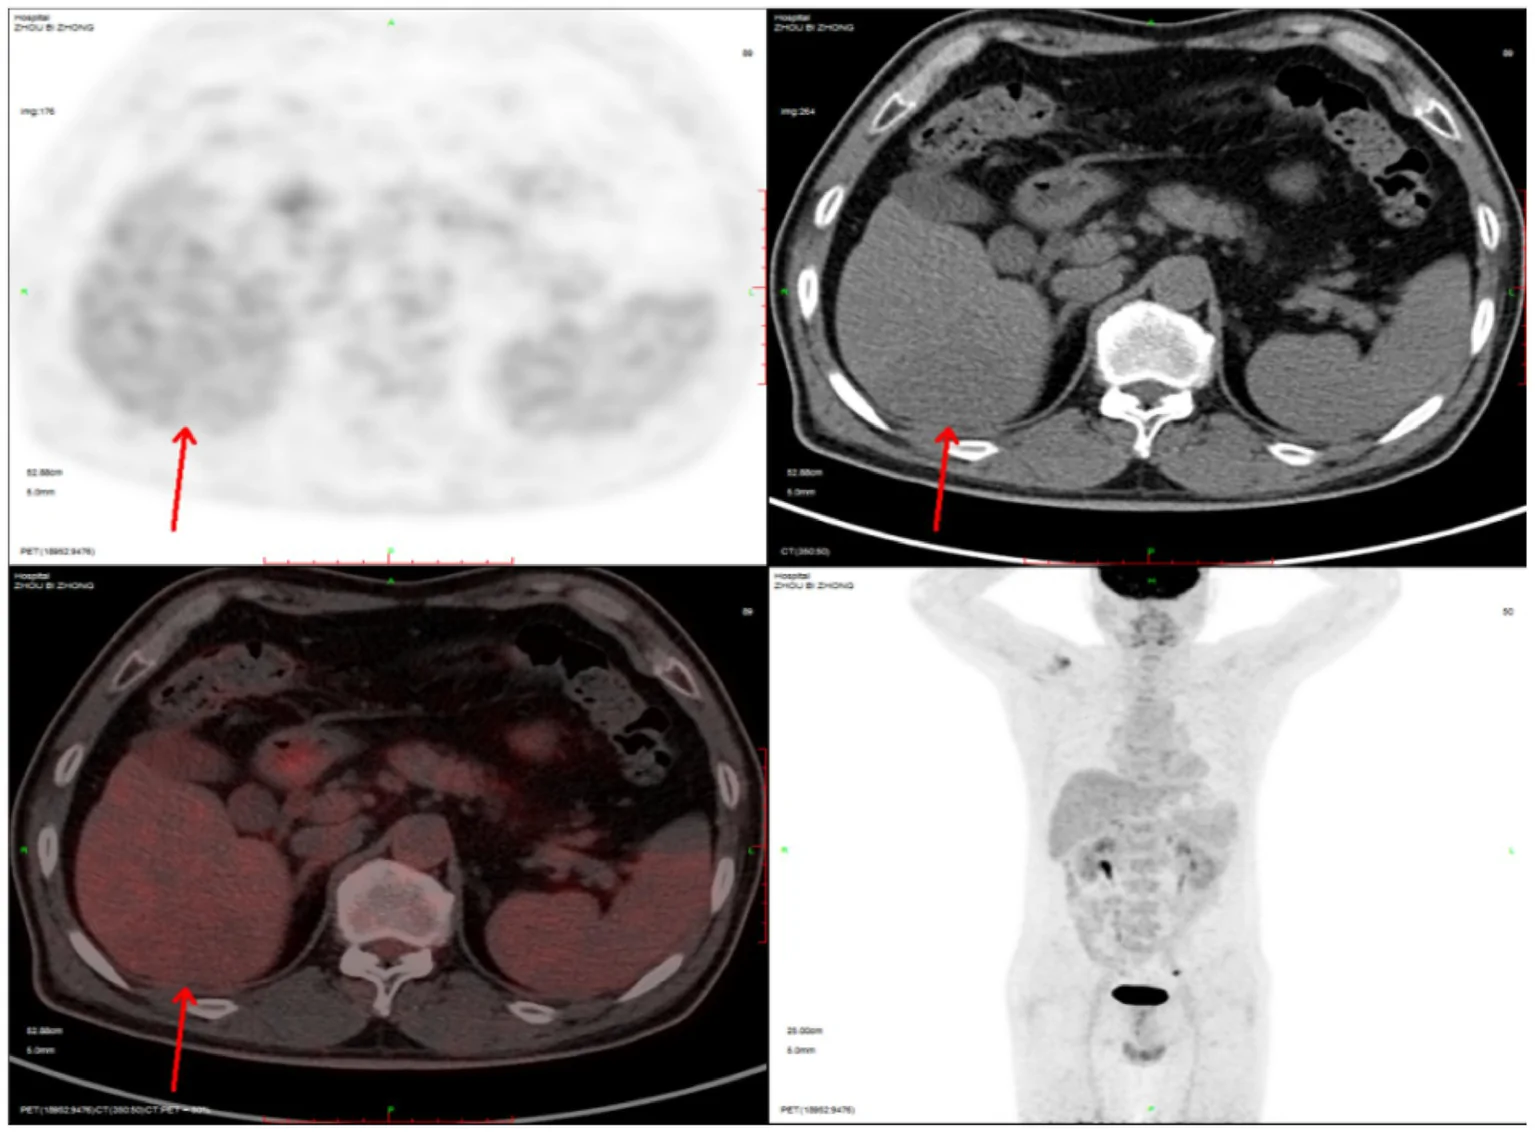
PET/CT: non-contrast CT reveals a nodular, mildly hypodense lesion in the posterior segment of the right lobe of the liver, with poorly defined borders. Mildly increased FDG uptake is noted (SUVmax = 3.1). The lesion measures approximately 5.8 × 3.7 cm.
